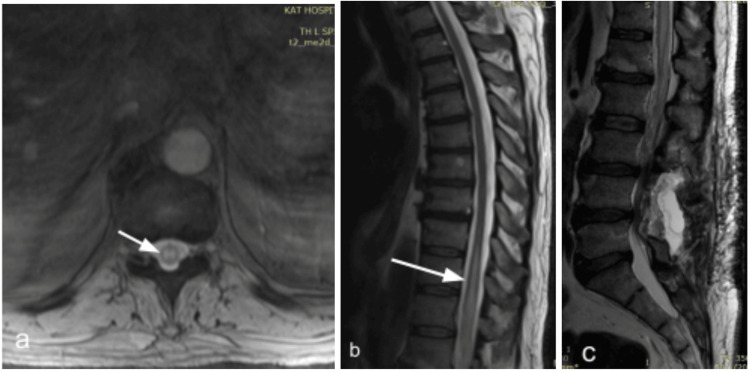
Postoperative MRI (second surgery) of patient #1. a: Persistent cord oedema in axial T2WI (arrow). b: same in sagittal T2WI. c: perimedullary venous plexus has been decongested.
